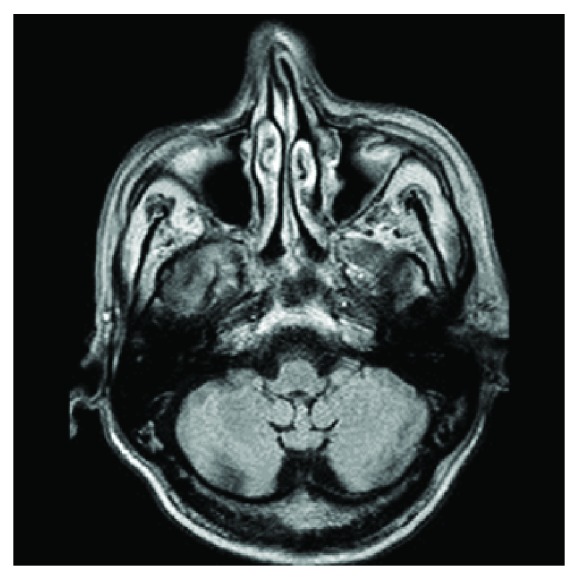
MRI scan 6 months after surgery.
